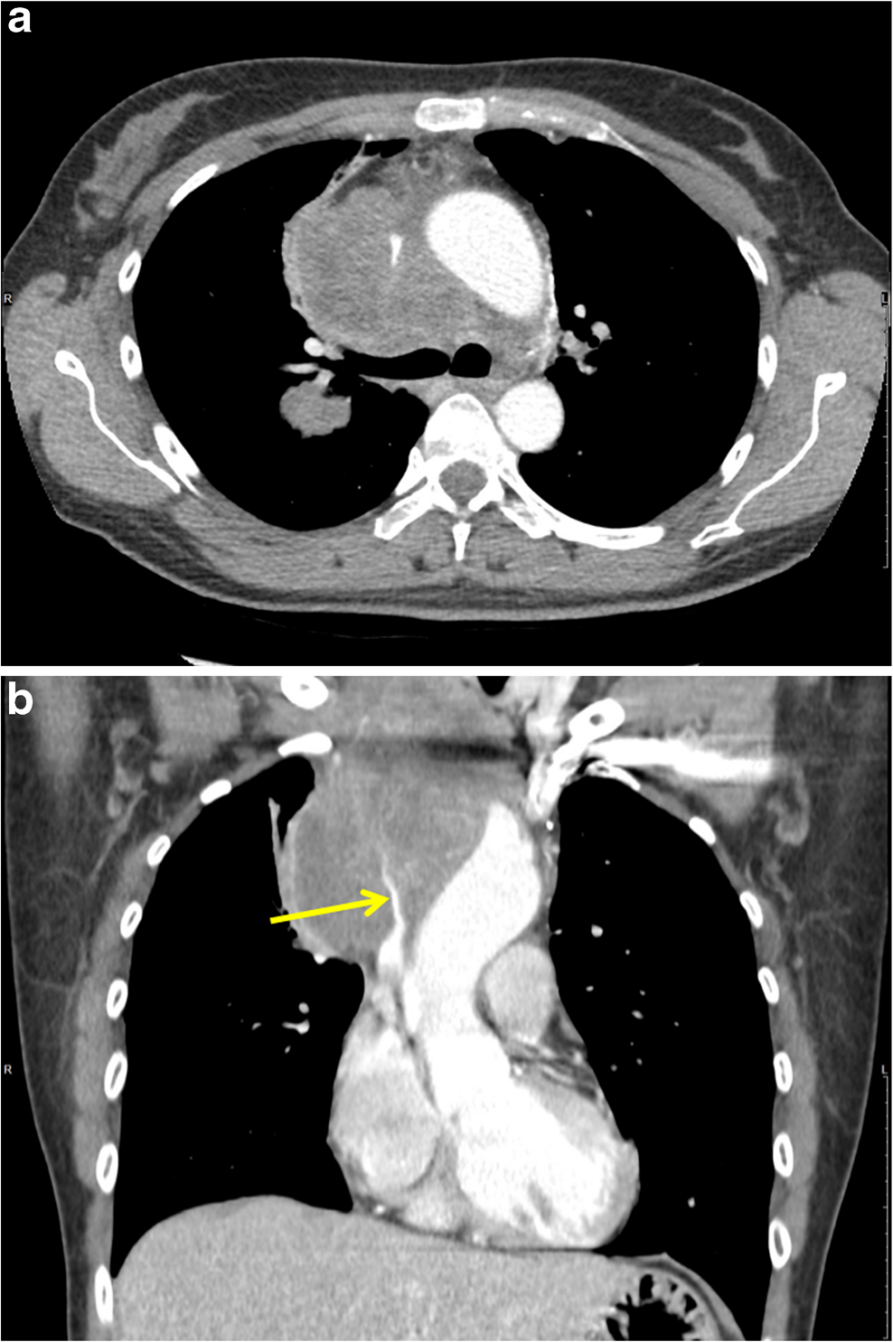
Superior vena cava syndrome (SVCS) as the initial presentation of a stage IIIB small cell lung cancer in a 48-year-old woman. a Contrast-enhanced axial CT image in mediastinal window setting depicts a large mass located in the visceral mediastinal compartment with encasement of mediastinum and in particular the SVC. b CT image reconstruction in the coronal plane shows the large soft tissue mass and better depicts the prominent narrowing of the SVC (*yellow arrow*)
